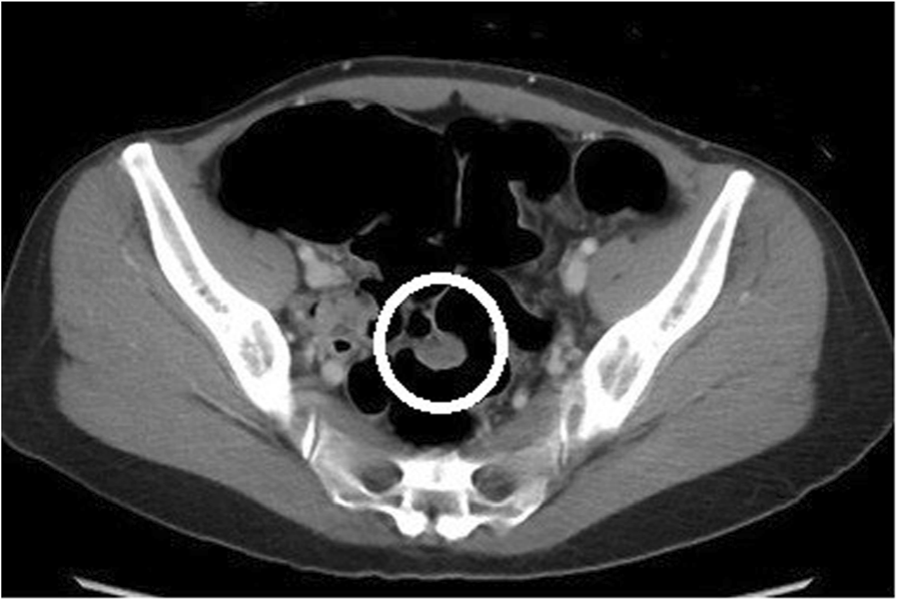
Computed tomography shows a 20-mm mass in the sigmoid colon (circle)
